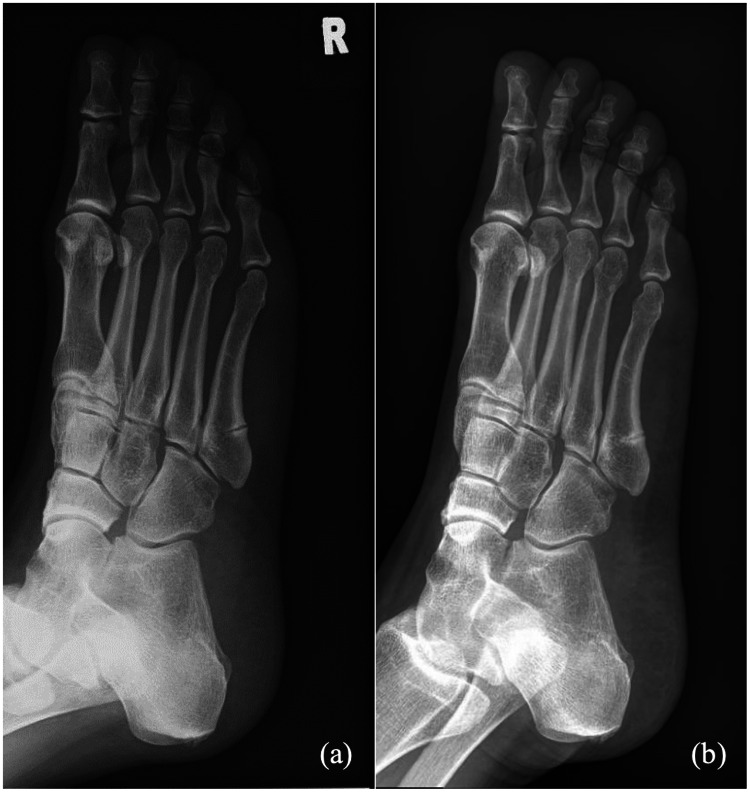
Radiographs of the right foot showing the Jones fracture in May 2023. (a) At initial presentation and (b) after six months
